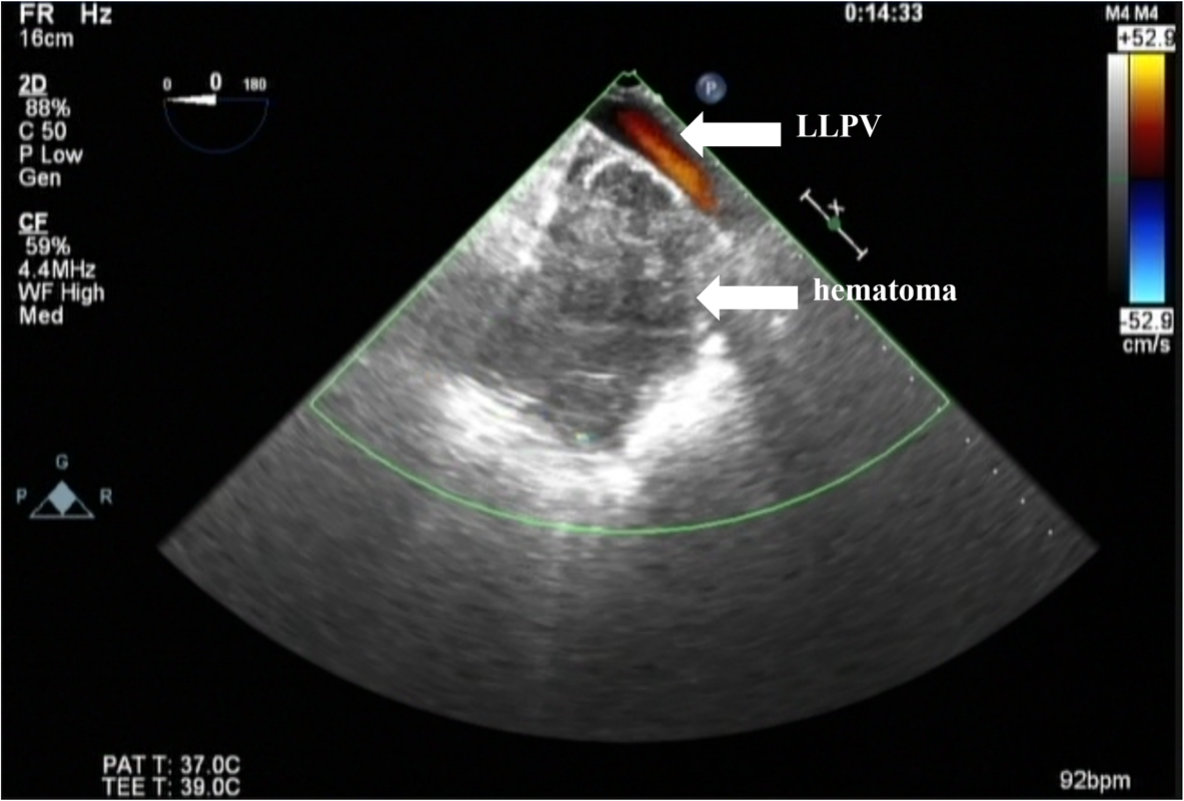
Left-sided intrathoracic hematoma adjacent to the left lower pulmonary vein area. Left lower pulmonary vein flow is depicted using the color Doppler imaging mode. LLPV, left lower pulmonary vein
